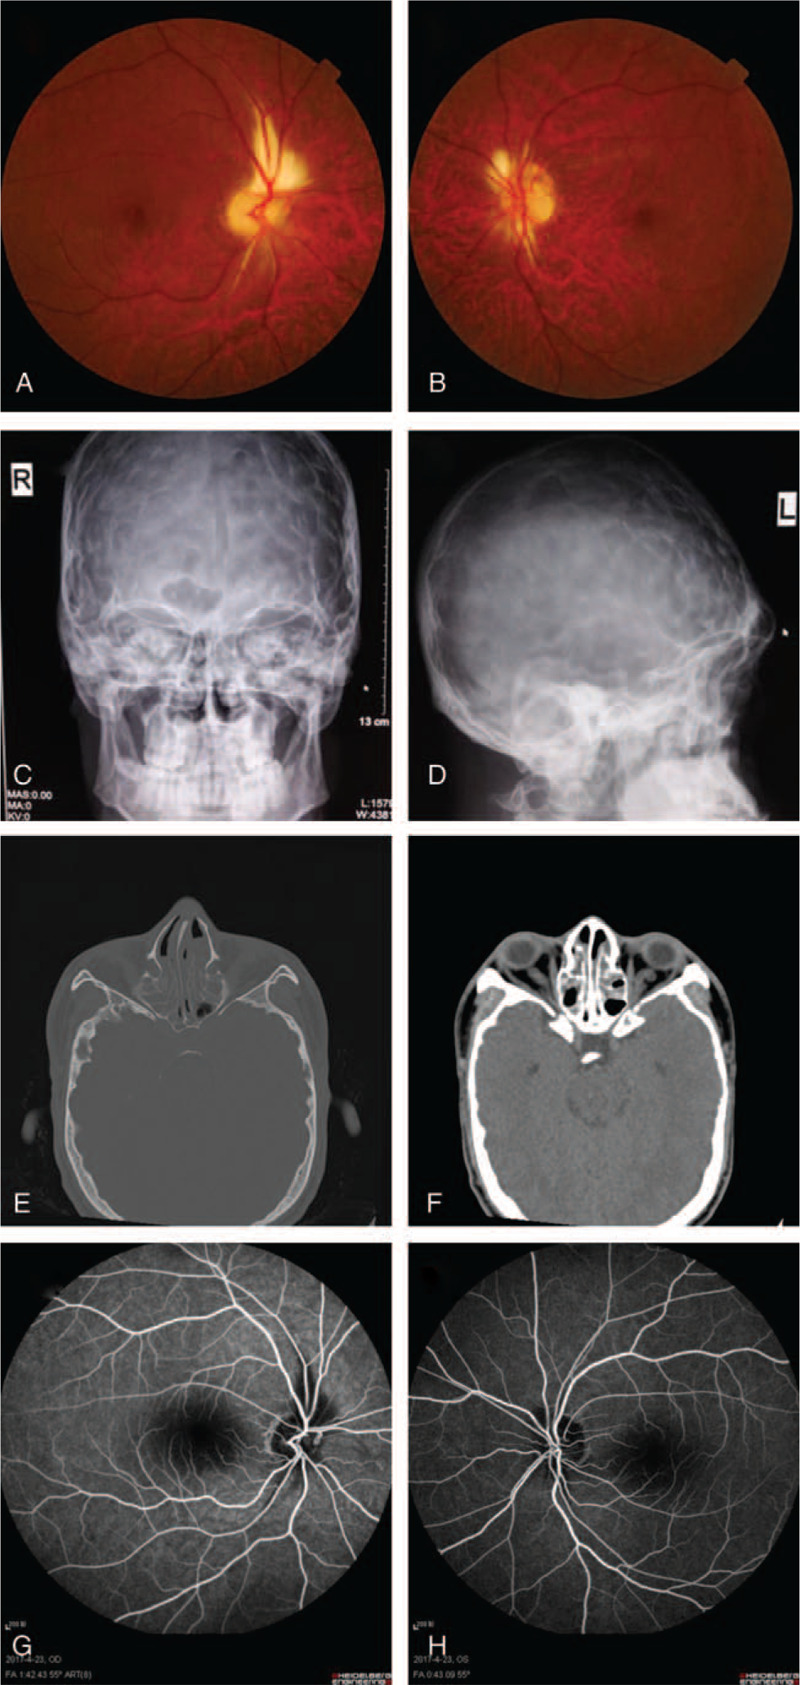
(A-B). Fundus photography of the proband's left (A) and right (B) eyes. (C-D) Cranial X-ray showed that distribution of the impressions gyrorum was seen deepening and widening in both bilateral skulls. (E-F) Orbital CT showed enlargement of medial rectus muscle in the right eye, and the optic nerve is tortuous in both eyes. Soft tissue density shadow can be seen in the intracavitary of the bilateral paranasal sinus. (G-H) FFA (fluorescein angiography) showed: binocular optic nerve atrophy, binocular retinal myelinated nerve fibers.
